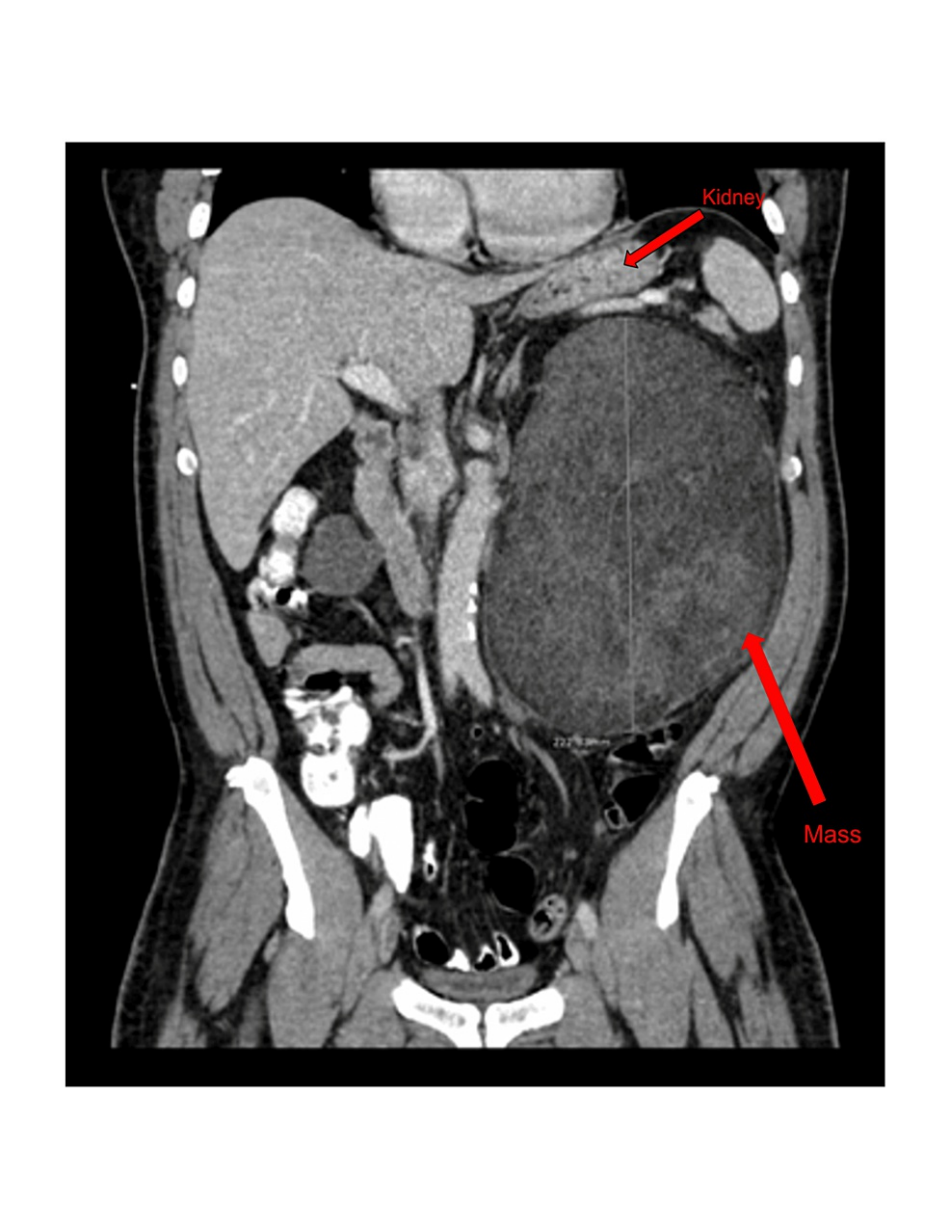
Patient imaging CT with IV contrast of abdomen in coronal view. Large mixed echogenic lesion measuring 22 x 13 cm in size with diffuse fat contribution and soft tissue elements suspicious for giant renal angiomyolipoma with mass effect but no definitive acute hemorrhage CT: computerized tomography; IV: intravenous
